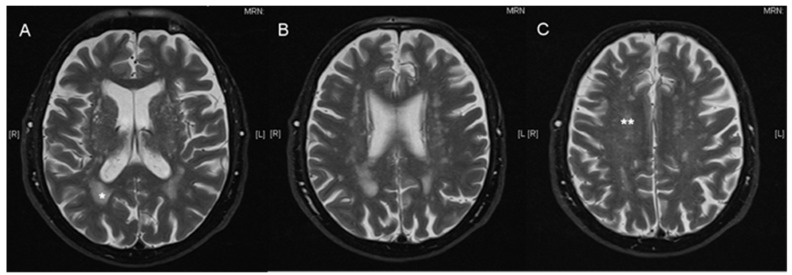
Exemplary MRI scan with annotated Fazekas score. Axial, T2 weighted cerebral MRI scan of an 87-year-old male patient before cochlear implantation with a Fazekas total score of 5, one asterisk indicates periventricular white matter lesions (PVWM *) score of 2; two asterisks indicate deep white matter lesions (DWM ) score of 3 (axial, A–C).
